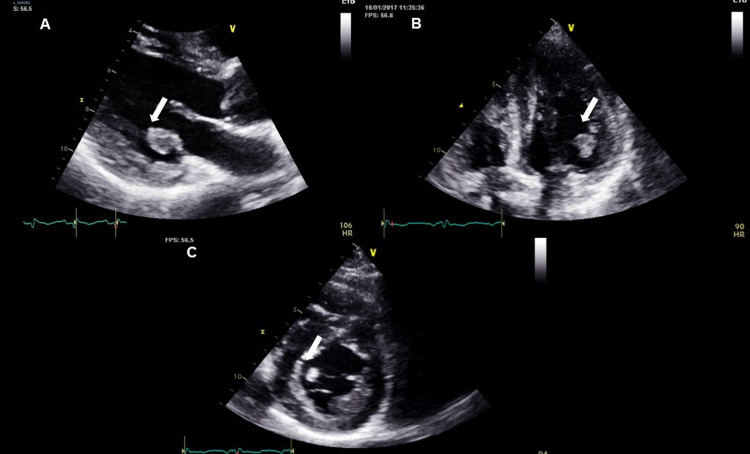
Aspergillus vegetation (arrow) of native mitral valve on echocardiogram A: Parasternal long axis view, B: Apical 4-chamber view, C: Parasternal short axis view
